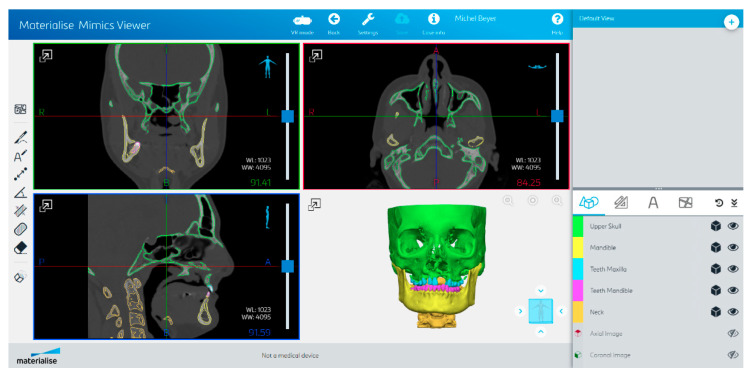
Materialise Viewer’s user interface (CT w/A 1 displayed).
